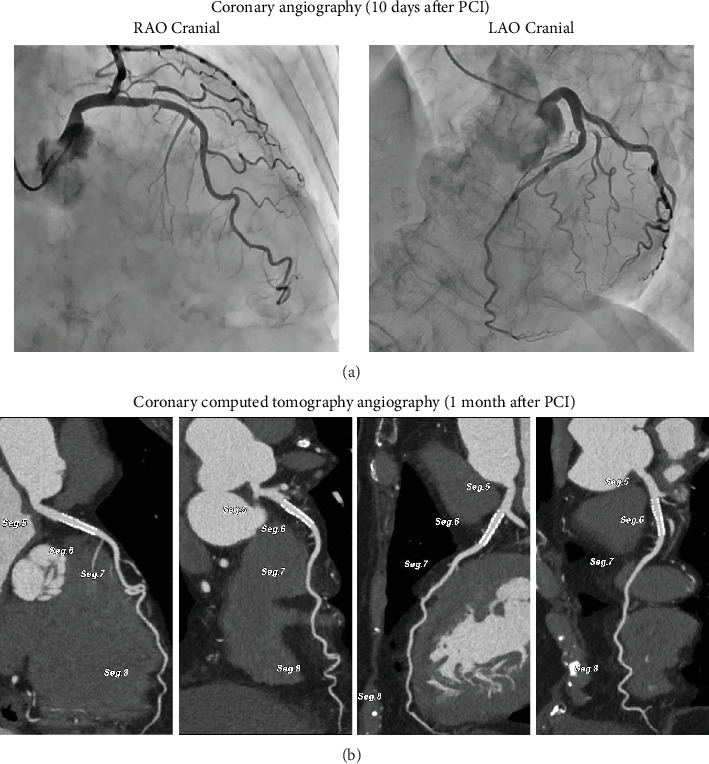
Coronary angiography and coronary computed tomography angiography. (a) Coronary angiography 10 days after percutaneous coronary intervention. Coronary angiography showing a tiny thrombus in the stent. (b) Coronary computed tomography angiography 1 month after the percutaneous coronary intervention. Coronary computed tomography angiography showing no thrombus in the left anterior descending artery.
